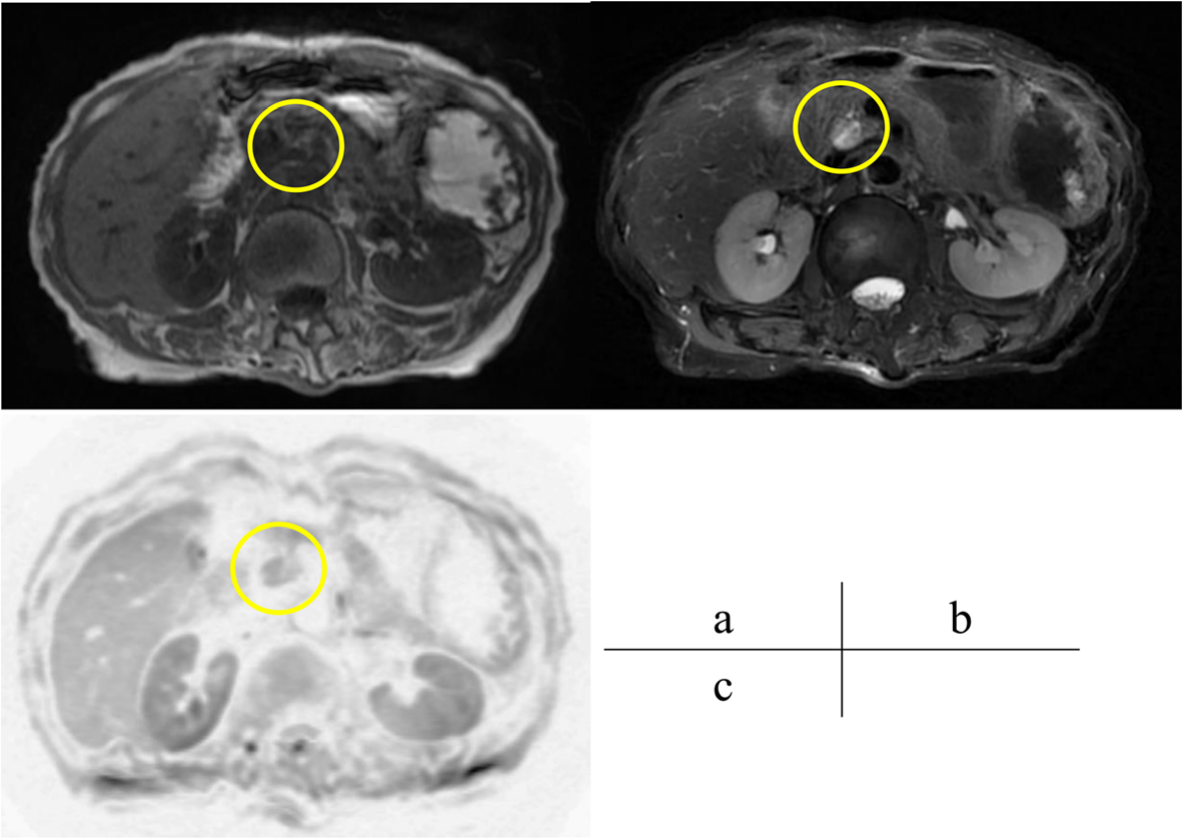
Magnetic resonance imaging (MRI) showing the patient’s tumor with low-intensity on T1-weighted images (a, yellow circle), heterogeneous high intensity (b) on T-2 weighted images, and high intensity on diffusion-weighted images (c)
